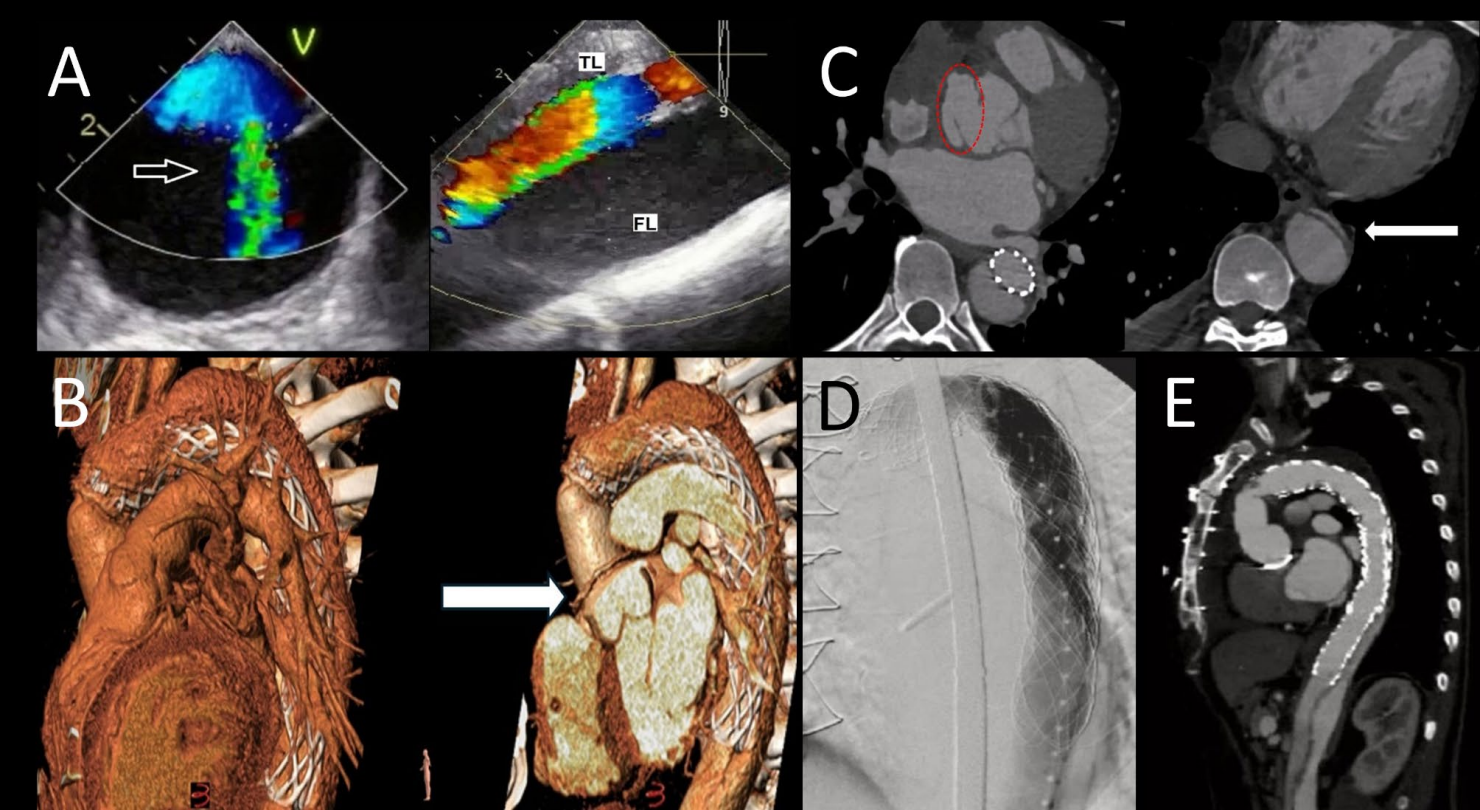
FET using E-Vita OPEN NEO trifurcated after failed AMDS as a redo surgery via re-sternotomy. (A) Transoesophageal echocardiography of descending aorta demonstrating a true lumen (TL) and an enlarged false lumen (FL) with maintained perfusion through re-entry (arrow). (B) Computer tomography (CT) 3D reconstruction showing aortic root pseudoaneurysm (arrow). (C) CT angiography (CTA) cross-sectional revealing pseudoaneurysm at aortic root (red circle) and subtotal true lumen collapse (arrow) at the descending aorta. (D) Intraoperative fluoroscopy displaying excellent E-Vita OPEN NEO Trifurcated implantation with stent-graft extension. (E) CTA control after 6 months showing patent aortic arch, E-Vita OPEN NEO with stent-graft extension, and obliterated FL with partial thrombosis
